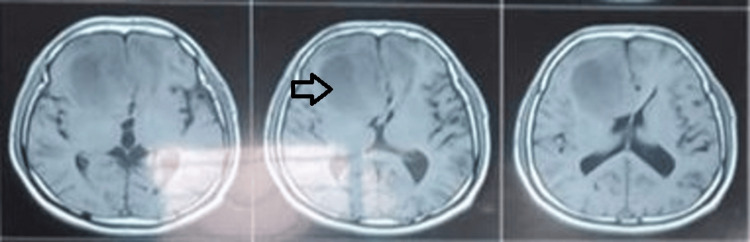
Depicting pre-operative MRI brain (T1 images); isointense intra-axial space-occupying lesion in the right frontal lobe.
